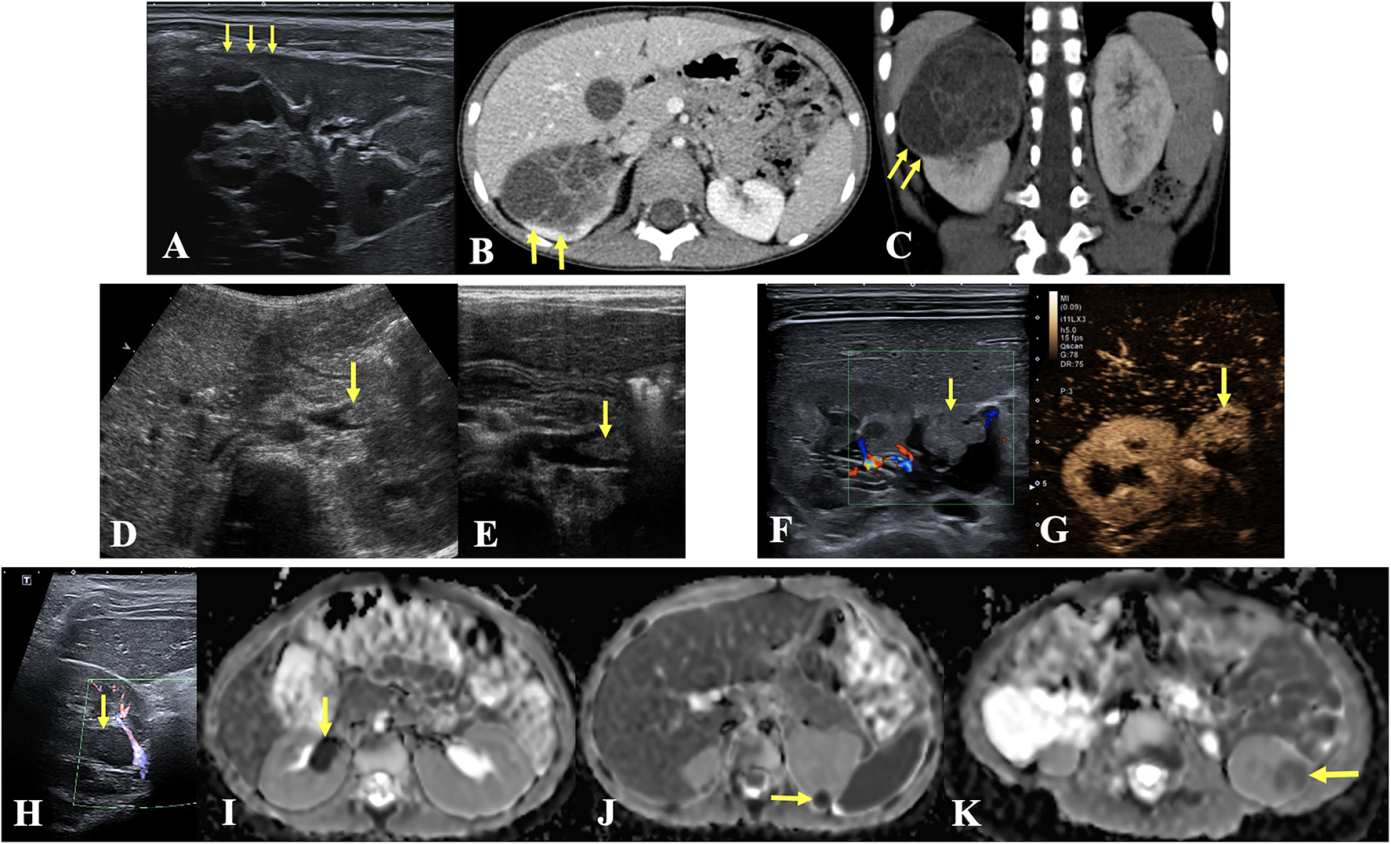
A–C Four-year-old boy involved in a car accident. Incidental finding on a CT scan done in a trauma setting. US and repeat CT days later clearly demonstrate (yellow arrows) a smooth angle between a cystic tumour and the adjacent normal parenchyma. This claw sign indicates the renal origin of this tumour, which was confirmed on pathology to be a cystic nephroma. D, E Four-year-old girl. US workup of a large left nephroblastoma revealed a tumour thrombus within the left renal vein (yellow arrows) (D—convex probe; E—linear probe). F Fourteen-month-old boy with a renal mass with a mixed echotexture on a horseshoe kidney. No flow was detectable on Colour Doppler within the echogenic portion of the mass. G Contrast-enhanced US shows the vascularised aspect of the lesion and rules out thrombus/debris. H Three-year-old girl with known Beckwith–Wiedemann syndrome. Screening US revealed a hypoechoic perihilar mass within the right kidney. I–K On apparent diffusion coefficient maps, the right renal lesion is easily depicted, as well as two other lesions not visible on US in the left upper and lower kidney, in keeping with nephroblastomatosis vs Wilms tumour
